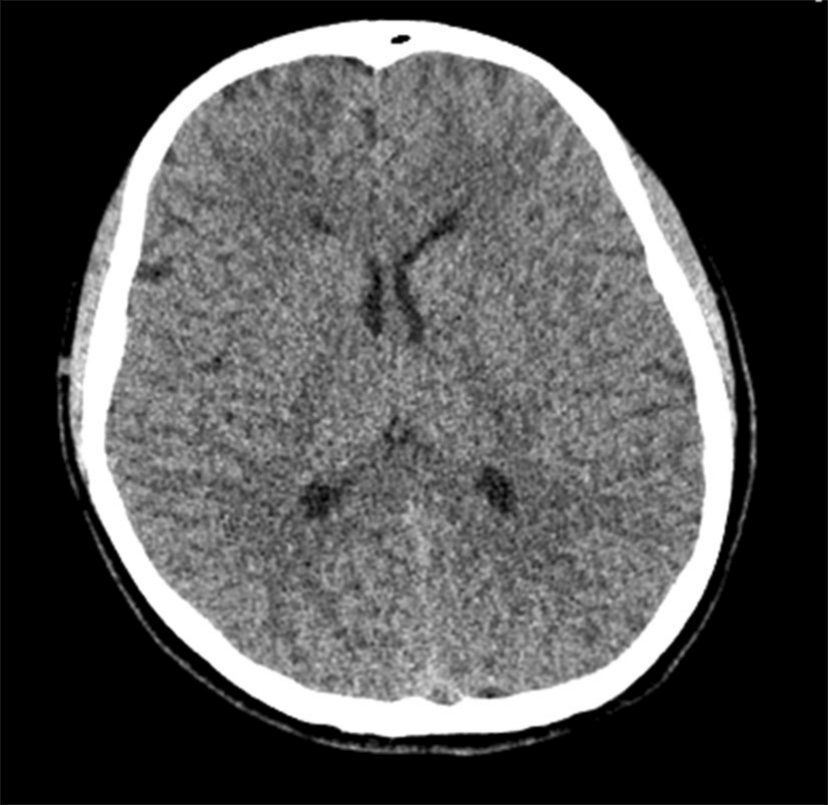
Patient’s brain computed tomography: no visible abnormality
